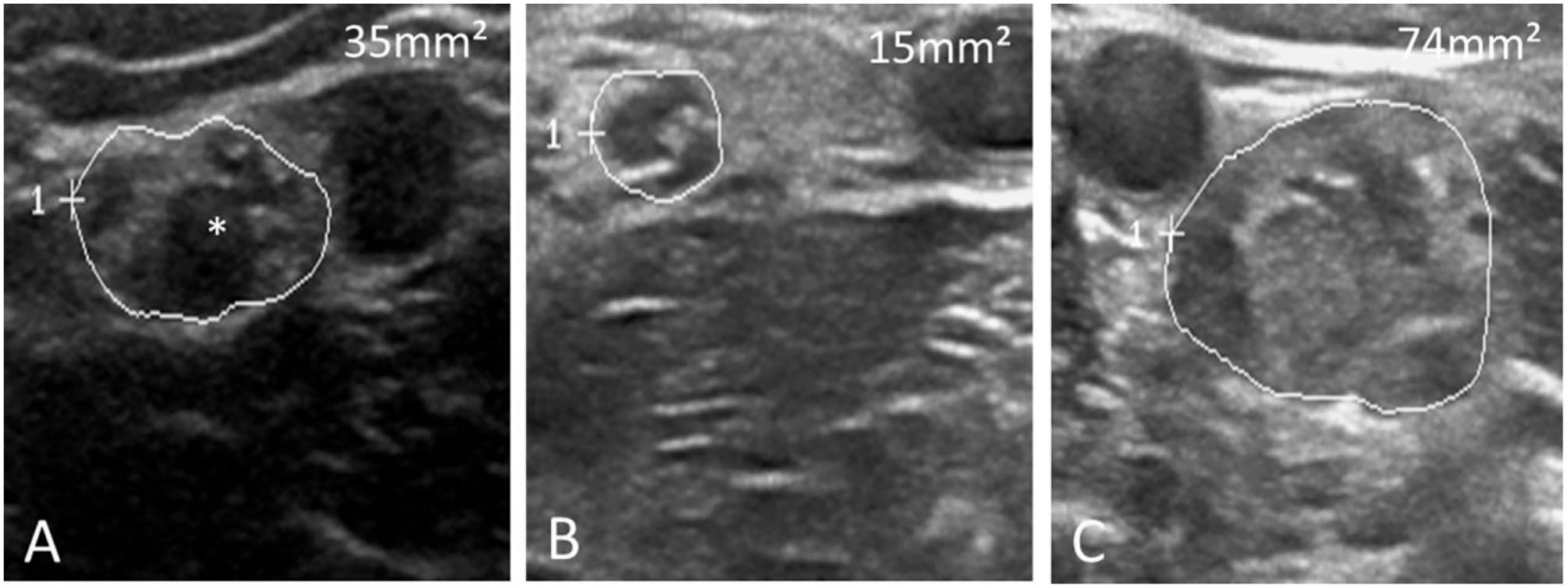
Three distinct ultrasound morphologies in chronic inflammatory demyelinating polyneuropathy. (A) Shows an enlarged median nerve (MN, CSA 35 mm^2^, normal values 13 mm^2^) with reduced echointensity and enlarged fascicles (*), while (B) Shows an only slightly enlarged nerve without fascicle enlargement (CSA 15 mm^2^). In contrast, in (C) the MN is tremendously enlarged (74 mm^2^) with otherwise hyperechoic intraneural echo signature. (A) Resembles the most common nerve morphology in chronic inflammatory demyelinating polyneuropathy.
